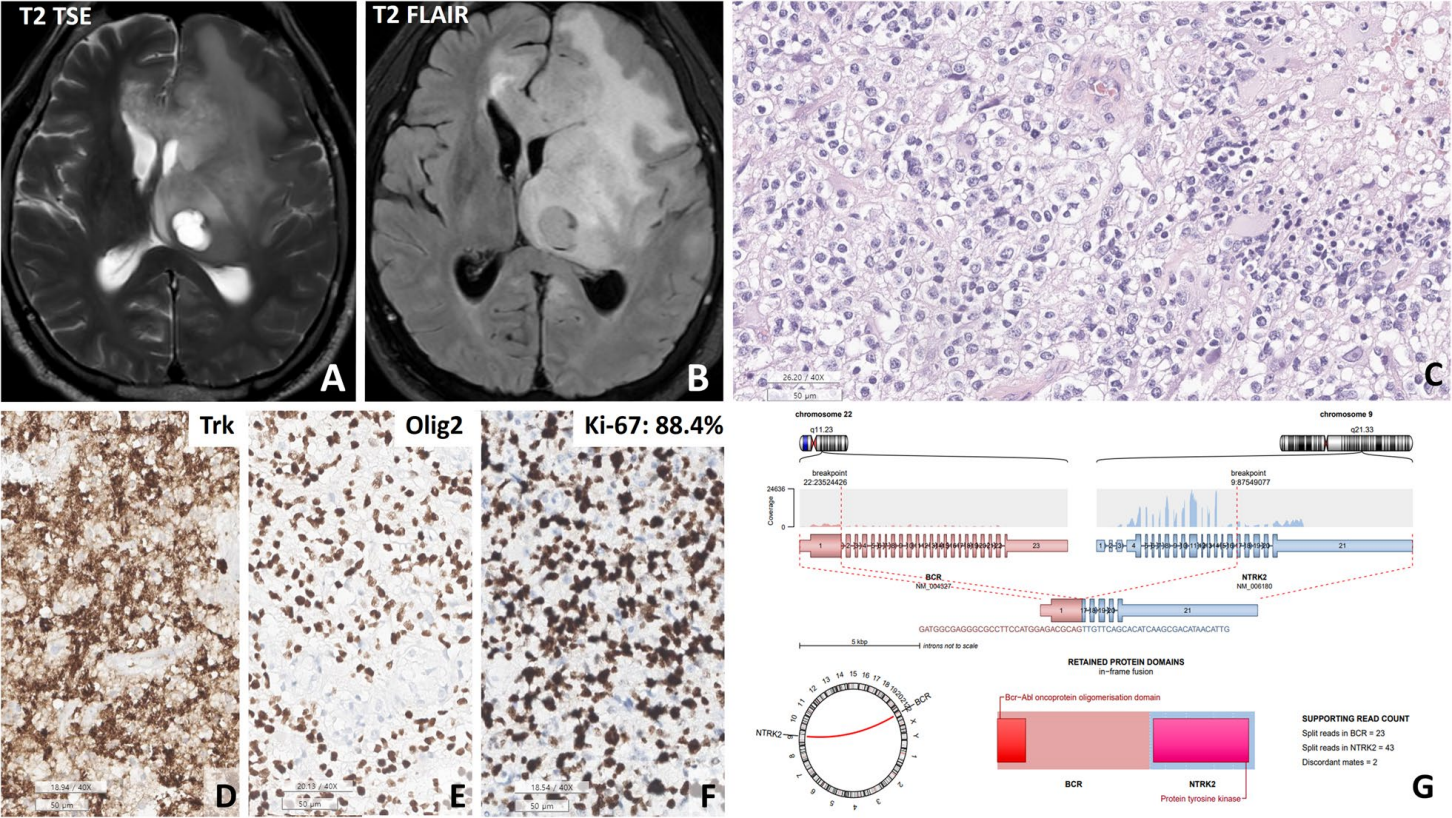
Patient #12 had the *BCR::NTRK2* fusion. A, B T2-weighted turbo spin‒echo (TSE) and T2 FLAIR images showing a hyperintense mass in the thalamus and bifrontal area with peritumoral oedema. C The tumour was composed of oligodendroglioma-like clear cells with microvascular proliferation and pseudopalisading necrosis. D and E Diffuse positivity for TRK and Olig2 was observed in the cytoplasm and nuclei, respectively, of tumour cells. F The Ki-67 labelling index was high (88.4%). G The Arriba plot revealed the in-frame fusion between the *BCR* gene and *NTRK2* gene (scale bar, F: 50 μm)
